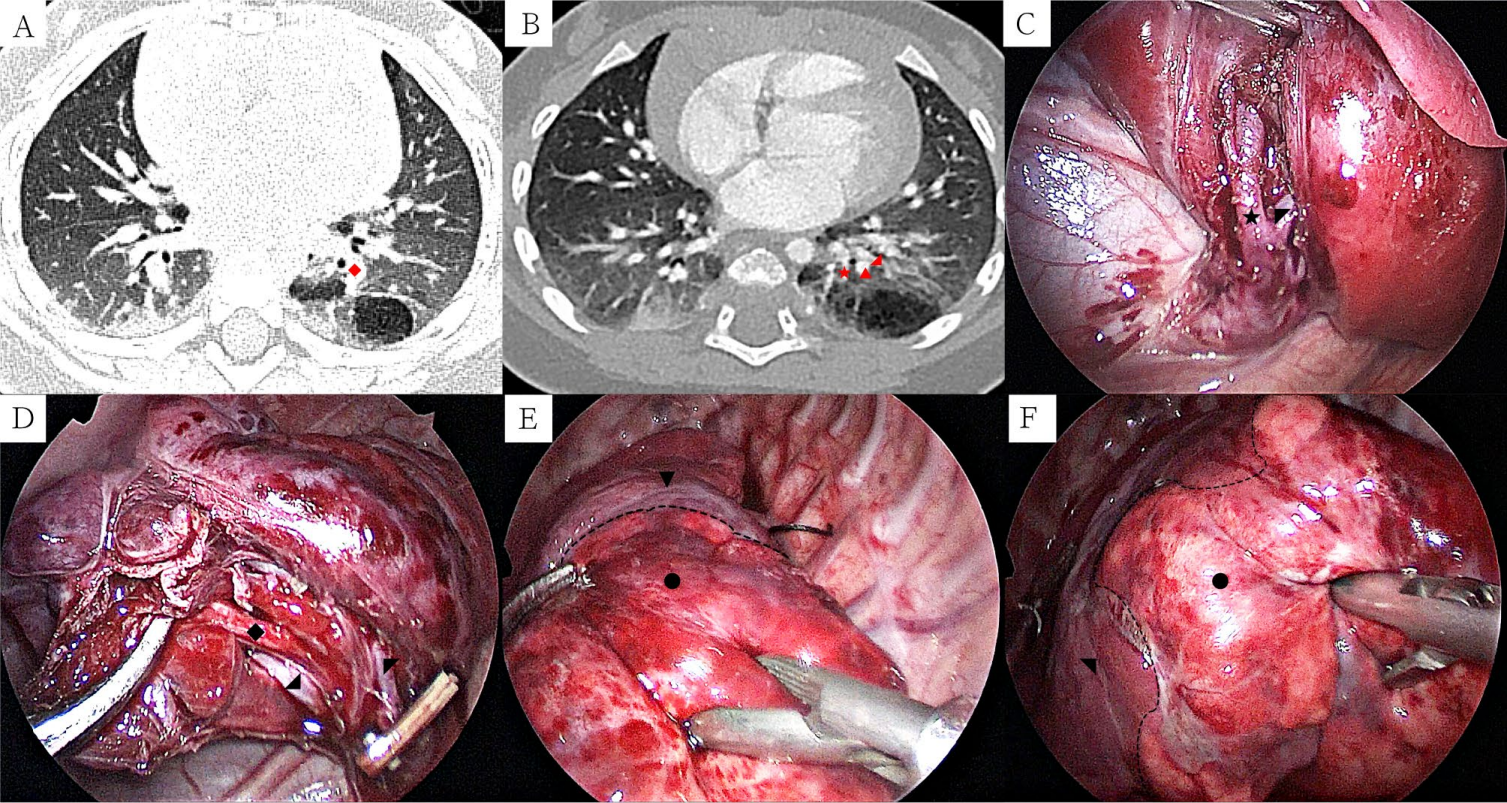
Preoperative CT and key surgical procedures of thoracoscopic segmentectomy of the left S10 via the inferior pulmonary ligament approach: (1 A) The lesion is located on the left S10, and B10 enters the lesion (lung window); (1B) Important structures that need to be cut off in left S10: B10, A10, V10, and V9 located at the boundaries of the lesion can be preserved (mediastinal window); (1 C) Separate the lower pulmonary vein and expose V10 and V6; (1D) After cutting off V10, separate and expose B10 from V9 and V6; (1E) Modified “expansion and collapse method” to determine the plane between segments, showing the boundary between S6 and S10; (1 F) Modified “expansion and collapse method” to determine the plane between segments, indicating the boundary between S9 and S10. (S6: ▼, S10: ●, S9: ◥, B10: ◆, A10: ▲, V10: ★, V9: ◢, V6: ◤)
